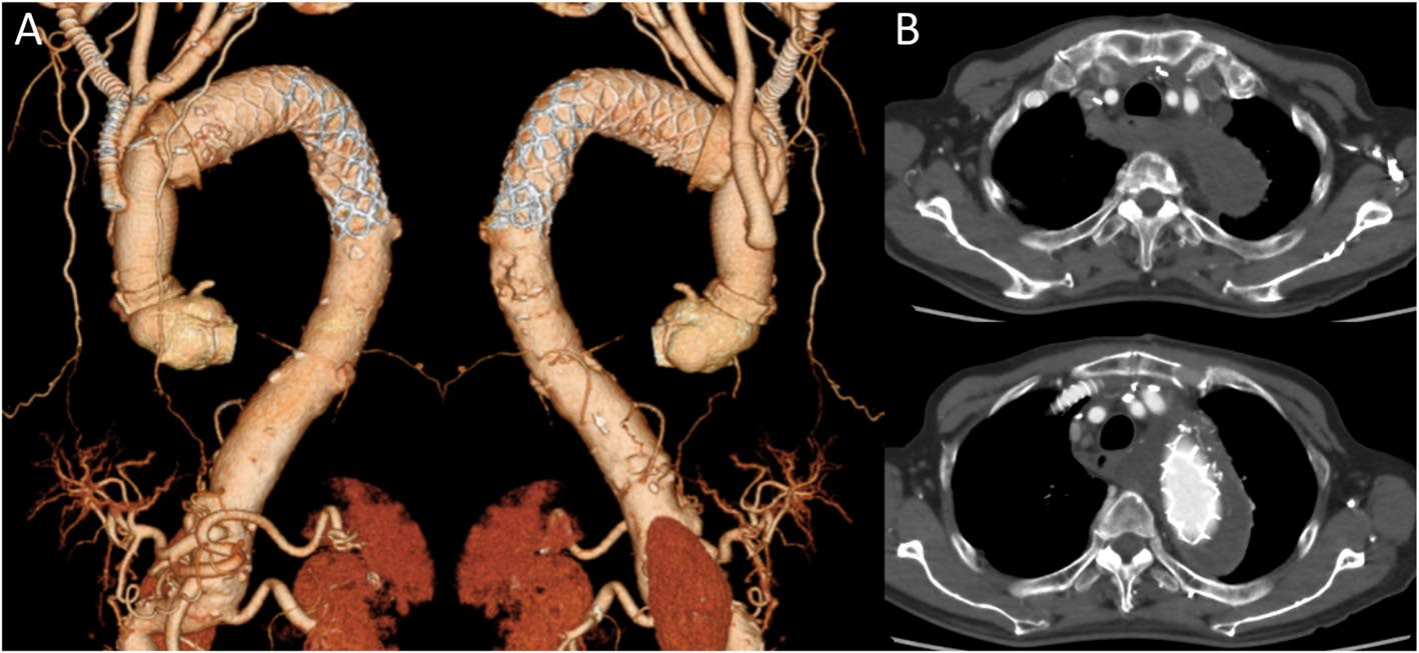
Postoperative computer tomography (CT). A Three-dimensional CT images (anterior and posterior). The right subclavian artery was reconstructed using a ringed expanded polytetrafluoroethylene graft. B Axial images showed that the aberrant right subclavian artery and the distal arch aneurysm were completely thrombosed
